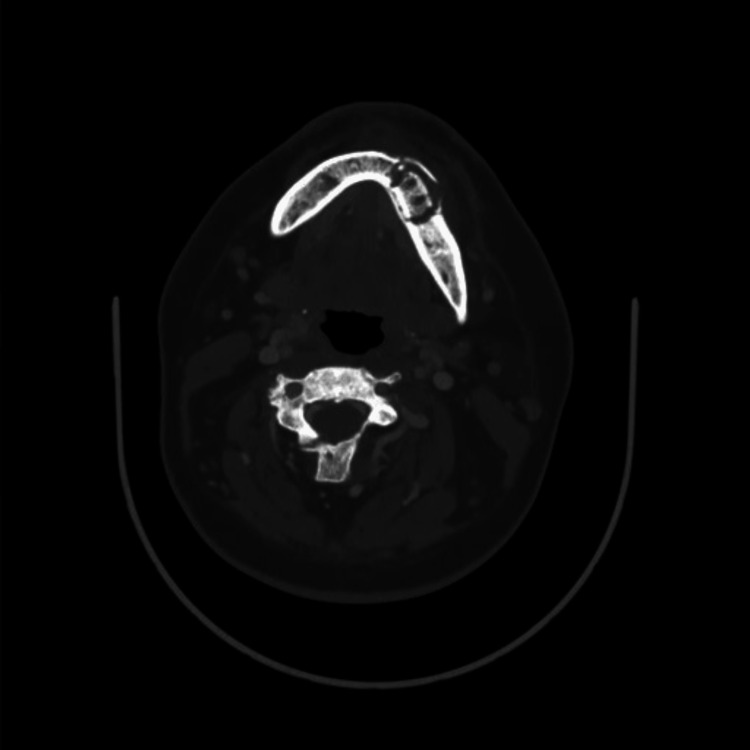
Axial CT Scan Depicting Mandibular Osteomyelitis in Osteopetrosis
